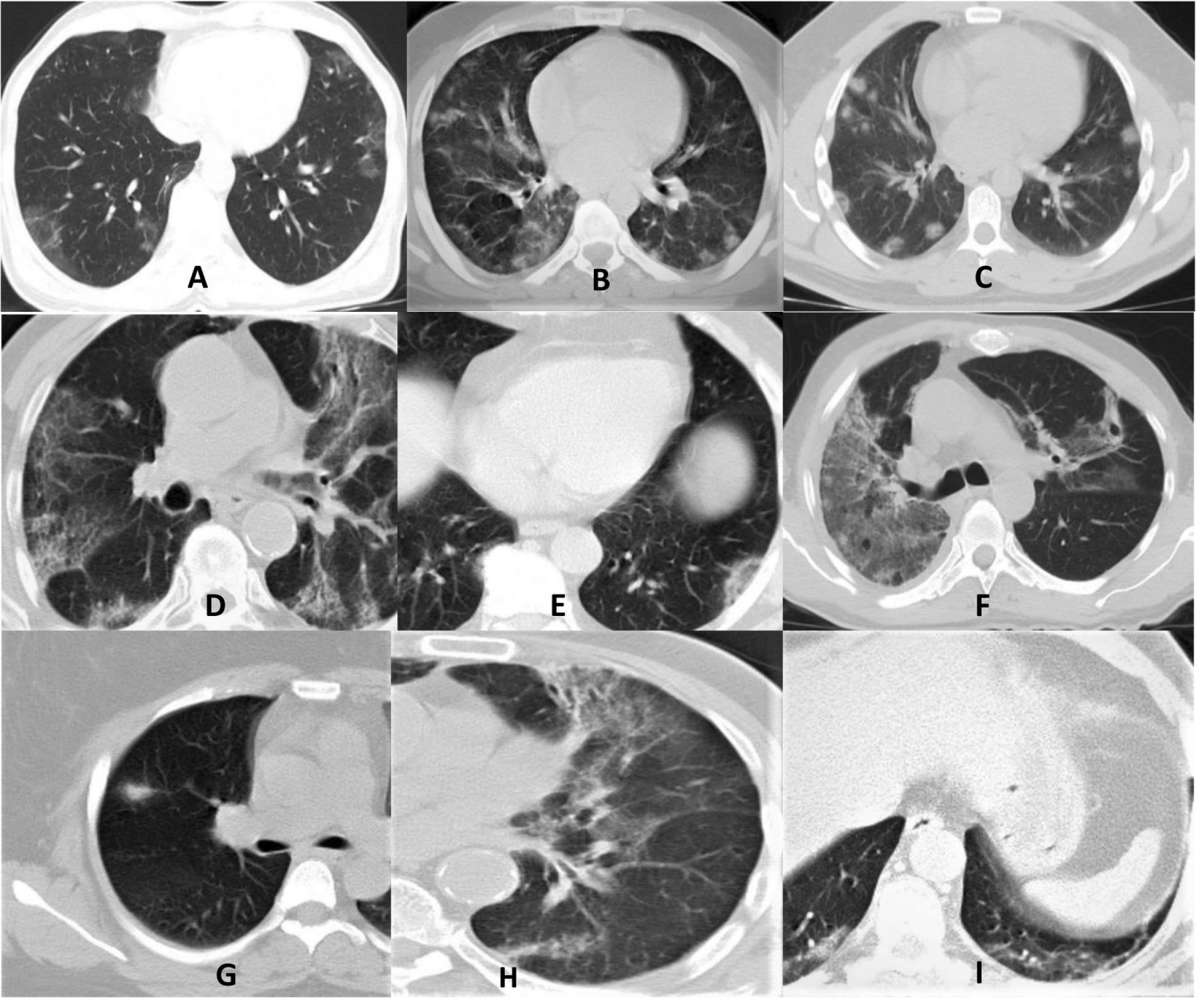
Lesion patterns on CT images of RT-PCR (+) patients. a GGO, b GGO + consolidation, c Nodular consolidation, d Crazy paving, e reversed halo sign, e air bubble sign, g halo sign, h airway changes, i curvilinear line
